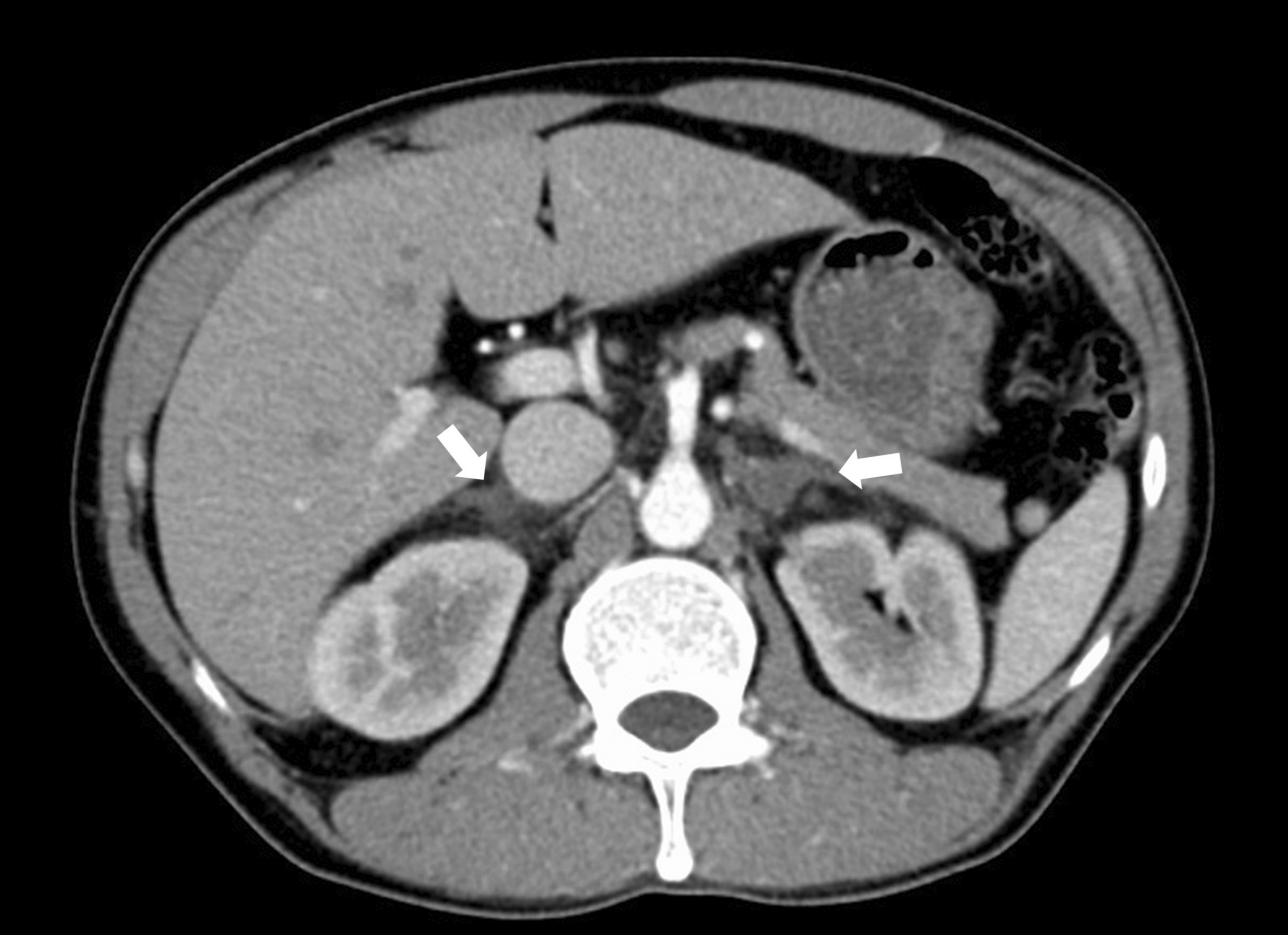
Thoracoabdominal computed tomography (CT) with contrast enhancement on admission. Imaging revealed diffusely enlarged bilateral adrenal glands with diffuse internal low‐attenuation areas and increased CT value in the surrounding adipose tissue (arrows).
